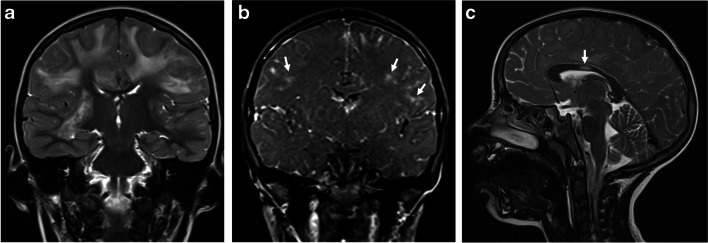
Magnetic resonance images show a leukodystrophy-like pattern in a 4-year-old girl with myelin oligodendrocyte glycoprotein antibody seropositivity, who presented with acute disseminated encephalomyelitis. a Coronal T2-weighted image shows bilateral confluent hyperintense signal changes involving asymmetrically the white matter. b Coronal fat-suppressed contrast-enhanced T1-weighted image shows bilateral nodular enhancement of the involved white matter (*arrows*). c Mid-sagittal T2-weighted image shows a small hyperintense lesion in the body of the corpus callosum (*arrow*)
